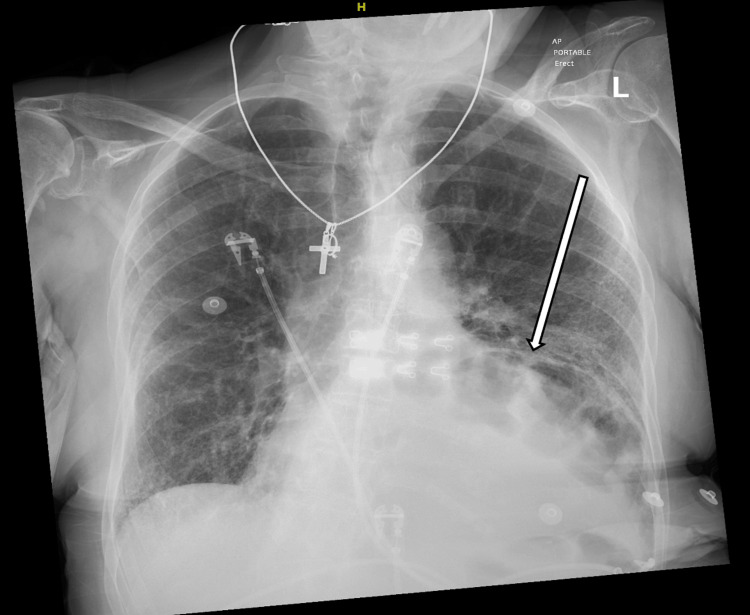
Posterior-Anterior Chest X-ray demonstrating bulging of the diaphragm on the left side and loops of bowel at the base of the left lung.
